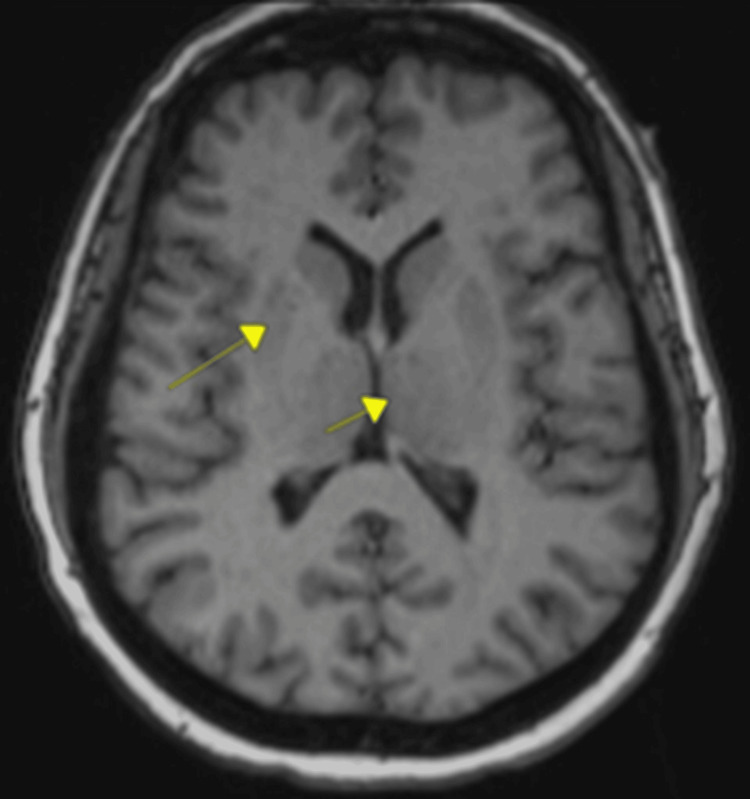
Axial T1-weighted MRI of the brain demonstrating bilateral symmetric hyperintensity within the globus pallidus (yellow arrows), consistent with manganese deposition MRI, magnetic resonance imaging
